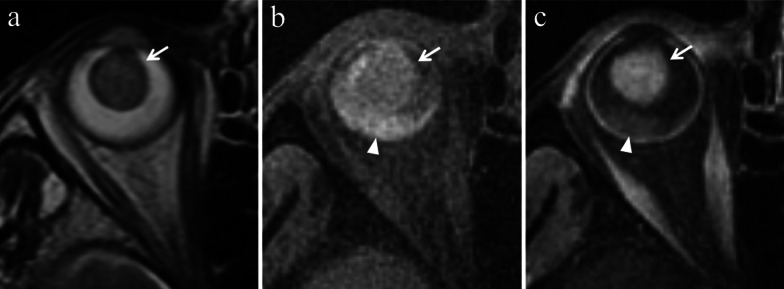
A 79-year-old woman with a poorly pigmented choroidal melanoma and hemorrhagic retinal detachment of the right eye. The same patient as in Fig. 8. Axial (a) T2-weighted turbo spin-echo STIR and (b) fat-suppressed T1-weighted images reveal an intraocular mass with intermediate signal intensity (white arrows), consistent with a poorly pigmented melanoma. Along the posterior aspect of the globe, the hemorrhagic retinal detachment is better depicted on fat-suppressed T1-weighted image in which it displays high signal intensity due to subacute blood products (white arrowhead). On (c) contrast-enhanced fat-suppressed T1-weighted image the lesion enhances (white arrow), whereas the retinal detachment does not enhance (white arrowhead)
